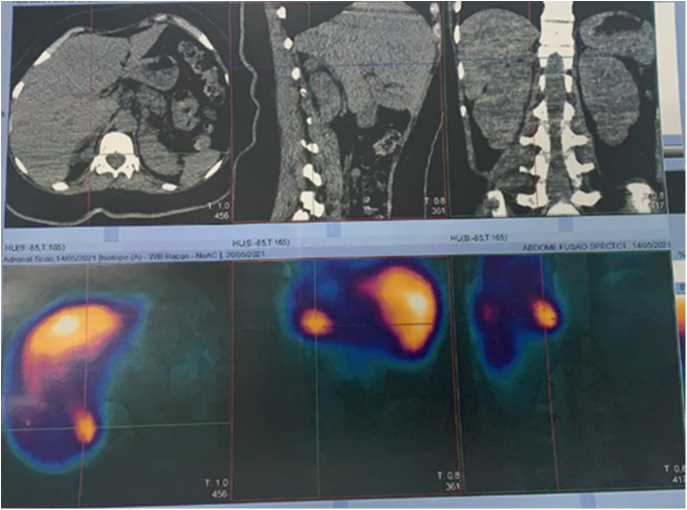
Abdominal single photon emission computed tomography with metaiodobenzylguanidine (MIBG) combined with computer tomography (SPECT-CT). We see increased radiotracer uptake in the topography of the right adrenal gland. From right to left, axial, lateral and coronal images.
